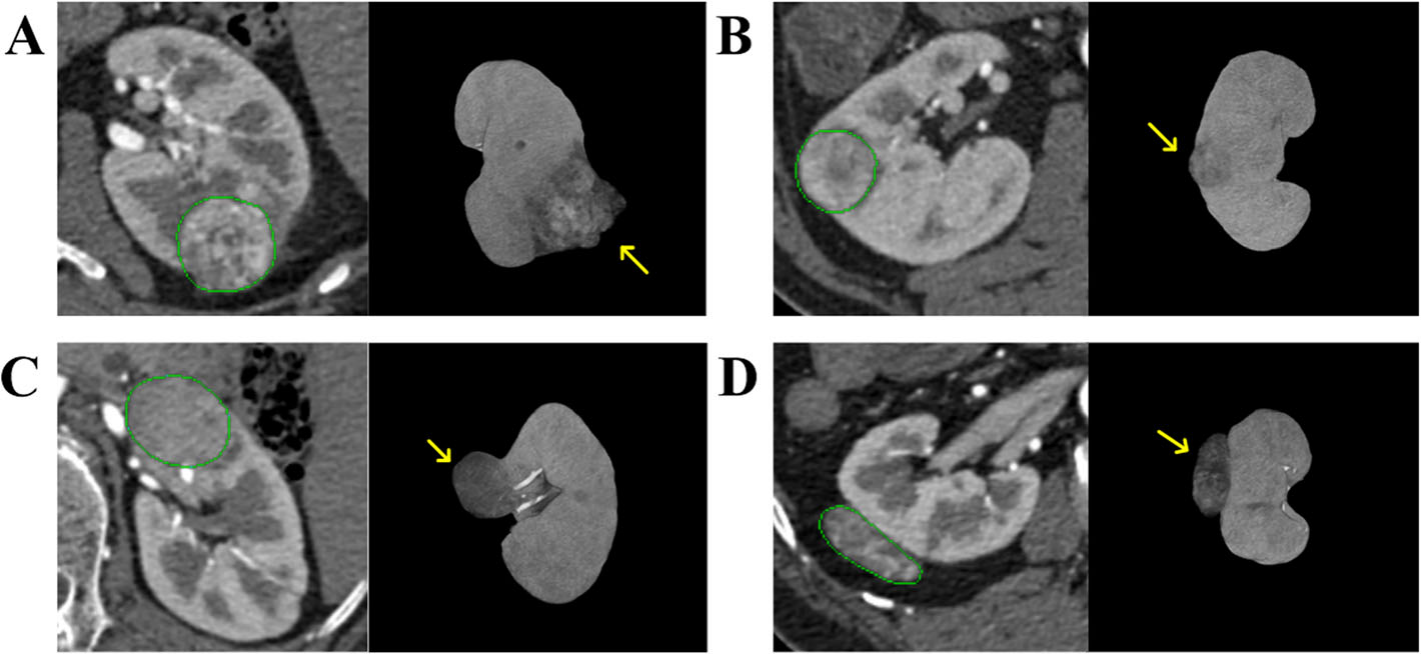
Four contrast-enhanced CT images of different pathological renal tumors. The tumors are marked by yellow arrows in 3D views. The manual contours of the renal tumors delineated by a radiologist are displayed in 2D slices. The pathological subtypes of the renal tumors are clear cell renal cell carcinoma (RCC) in (a) and (b), chromophobe RCC in (c) and angiomyolipoma in (d)
